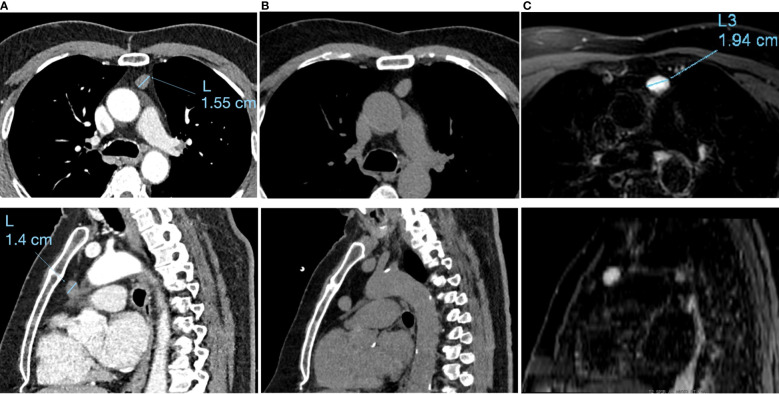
Timeline with relevant data from the episode: Axial incidences (upper range) and sagittal incidences (lower range) of the: (A) 2015 contrast-enhanced chest CT scan examination, (B) The 2021 native chest CT scan examination and (C) the July 2021 T2 sequence of the MRI examination.
